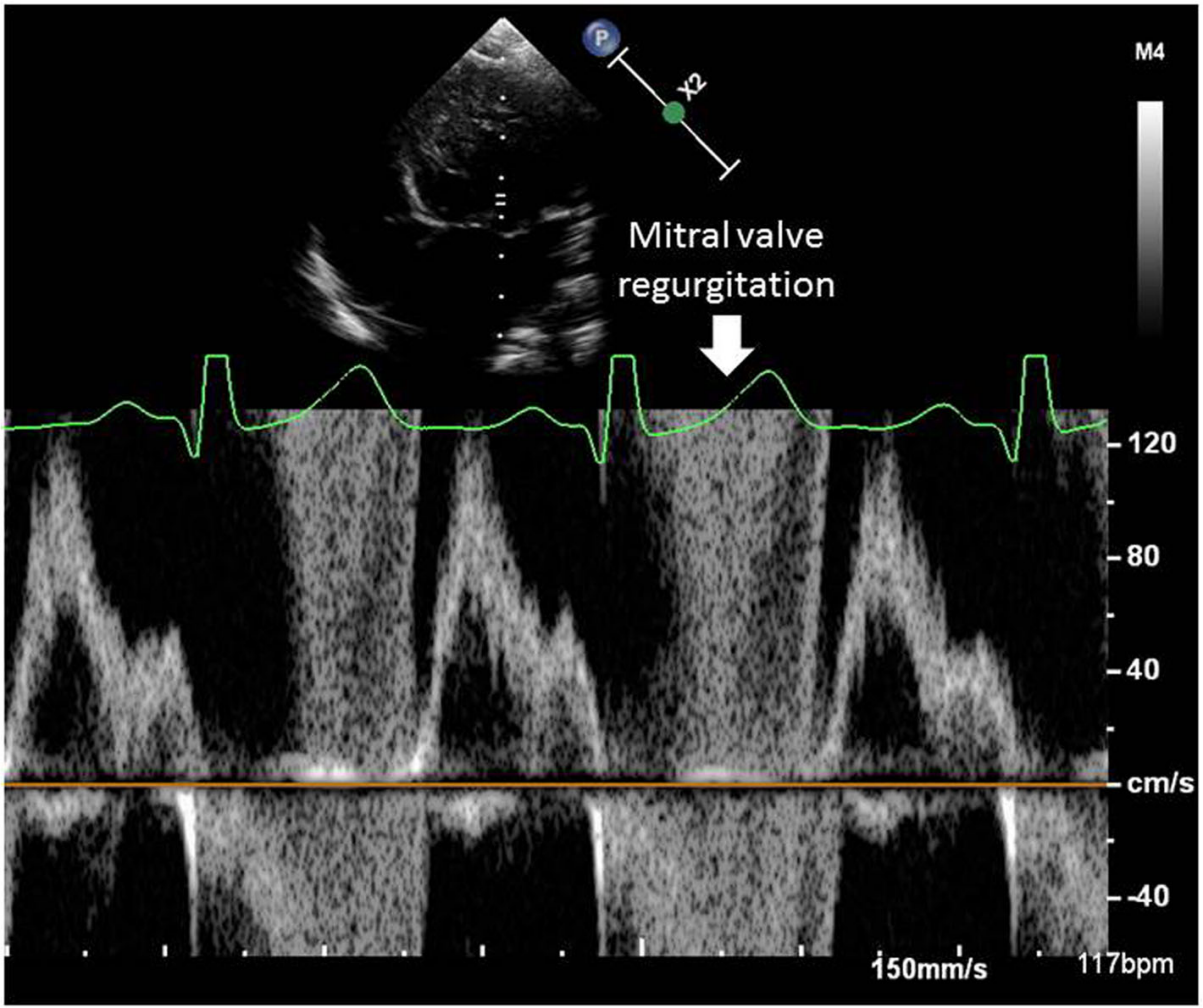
Apical 4 chamber view, focused on mitral valve. Notice the large mitral regurgitation jet on color Doppler. LV: left ventricle; LA: left atrium; MV: mitral valve
